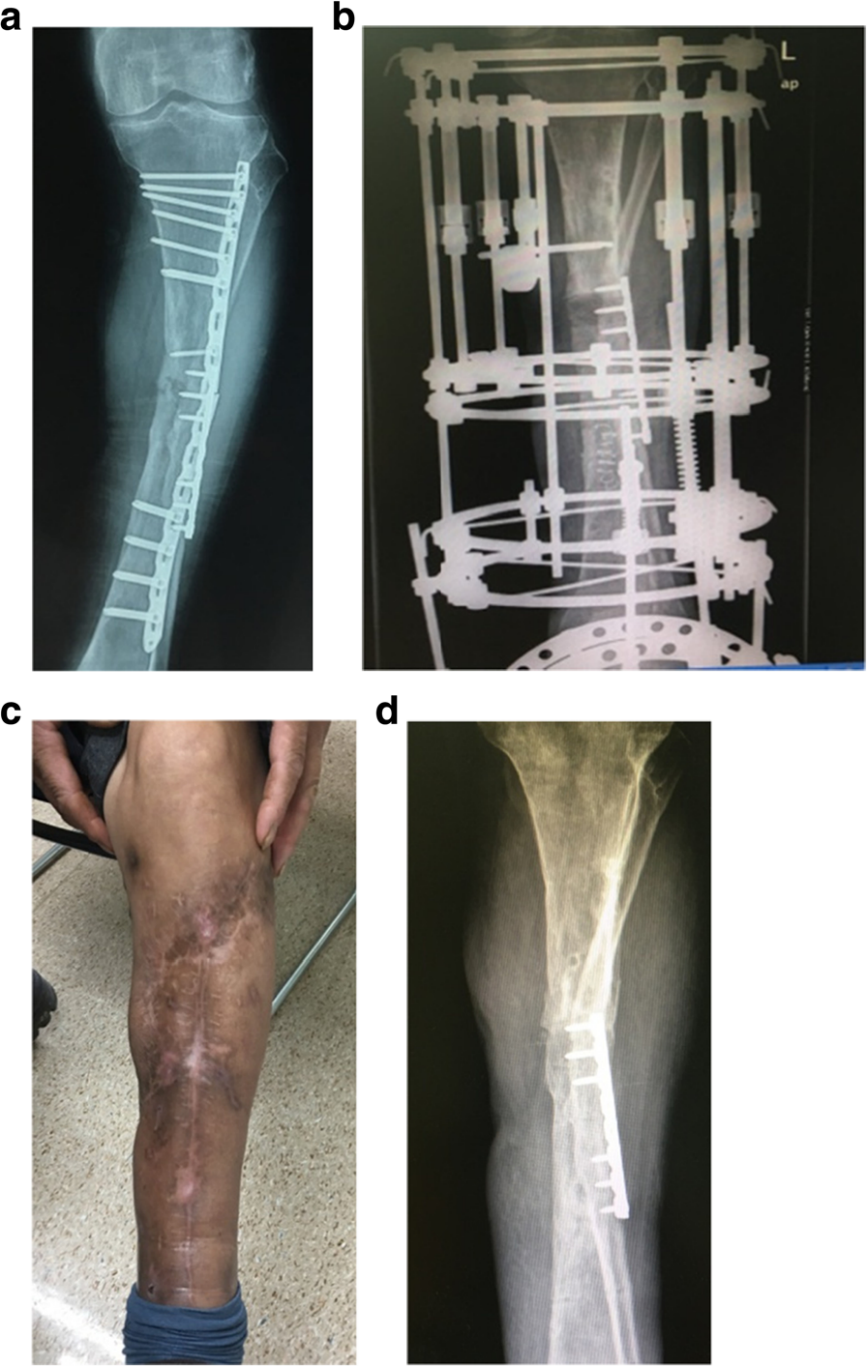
Unstable hardware + bone not healed = remove hardware, temporary stabilize/Ilizarov technique, debridement, soft-tissue coverage, and reconstruction when clean. a Radiographs of primary internal fixation. b The implant was removed and replaced by Ilizarov external fixation with segmental bone transport technique. c After 18 months of treatment, the wound was stable. d Radiograph 18 months after treatment
